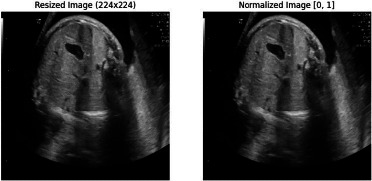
Comparison of resized and normalized ultrasound images for analysis.
